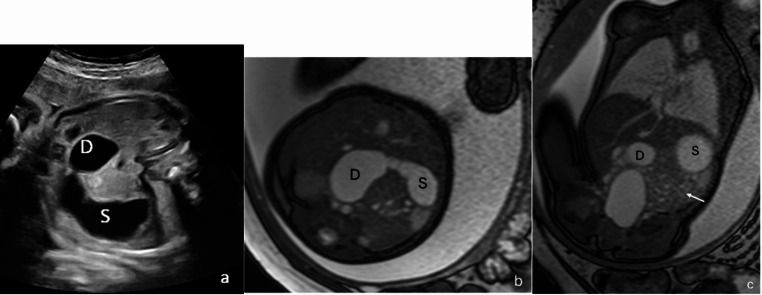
Prenatal imaging findings of duodenal atresia Transverse (a) screening US image at 23GW and transverse (b) and coronal (c) T2-W MR images at 30 GW show the classic “double bubble sign” with distended stomach (S) and proximal duodenum (D). Note increased amniotic fluid quantity in (b) and collapsed left bowel loops (arrow, c)
